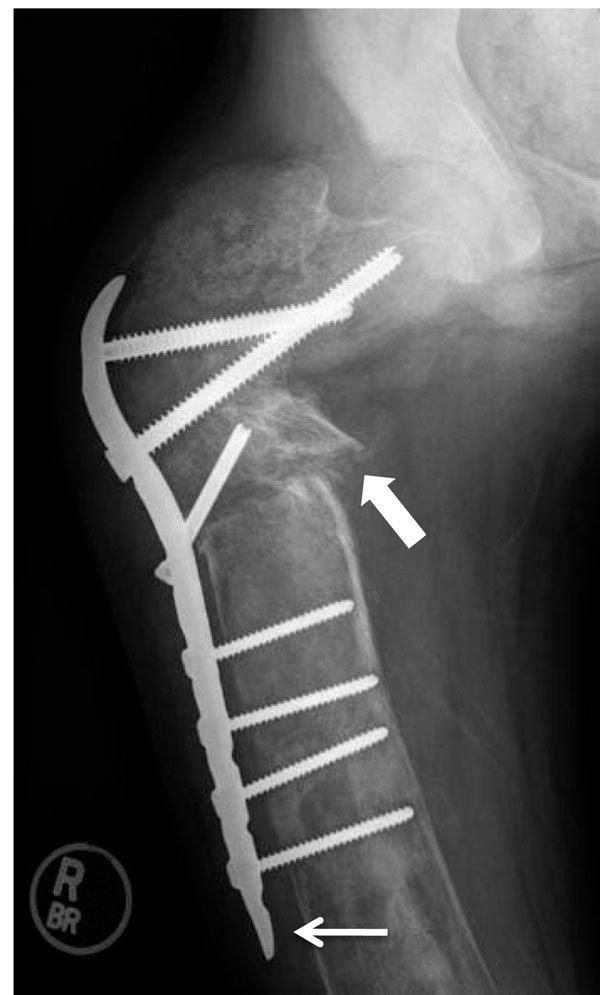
Screw and plate device in upper femur. This radiograph demonstrates the first stage reconstruction in a patient with severe deformity using a plate and screw device. The weak bone cortex results in poor fixation and eventual failure. Plate and screw constructs should be avoided if possible, or used only for temporary fixation until intramedullary fixation is possible. The thin white arrow demonstrates a problem typically encountered when fixation screws are inserted in to bone affected with FD, i.e. the plate has pulled away from the bone due to loss of screw fixation.
